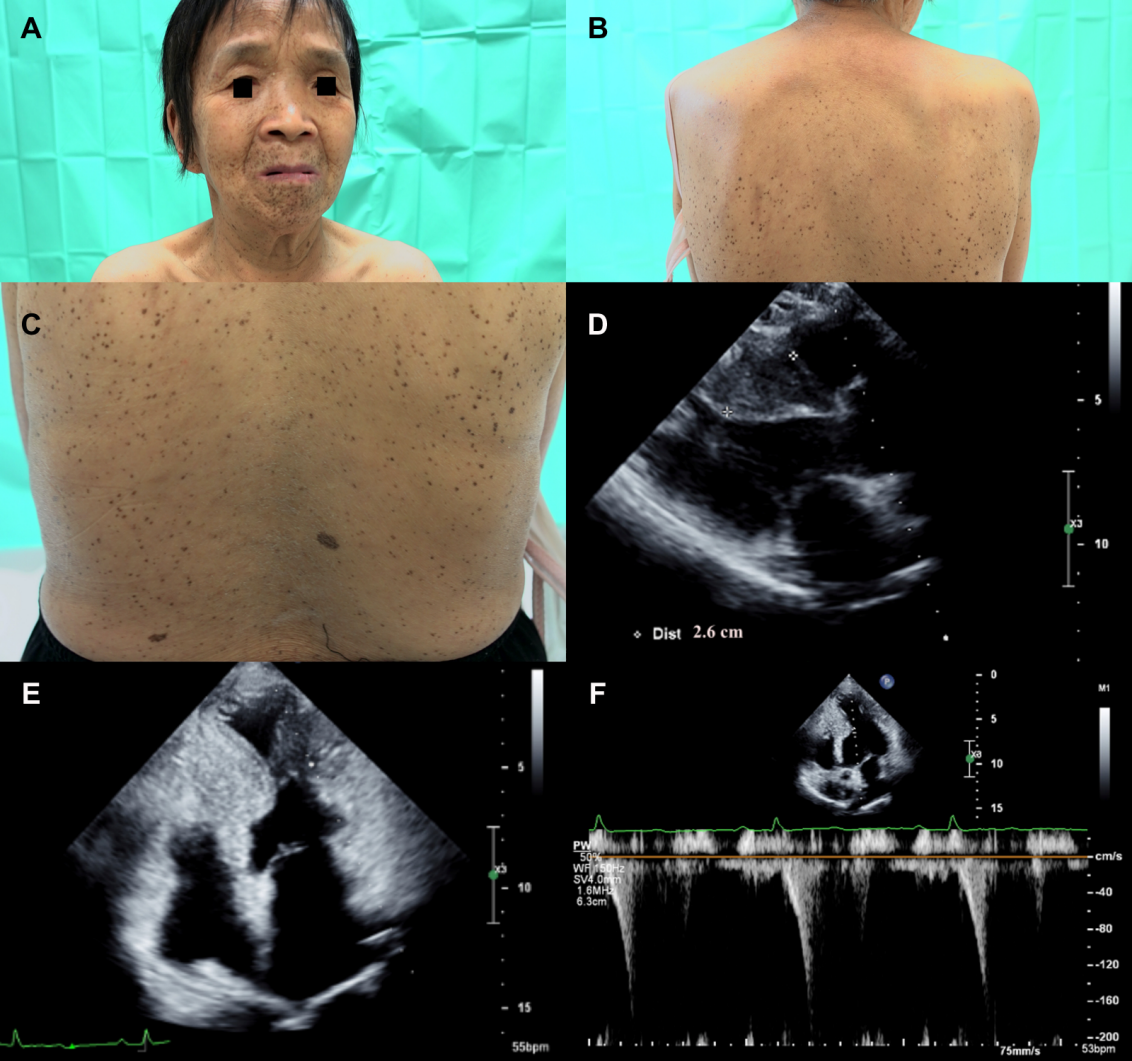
(A) The proband's mother (individual I-8) presented with ocular hypertelorism and diffuse black to brownish facial lentigines. (B) Numerous lentigines on her back, varying in size and shape. (C) Close-up of back lentigines with diameters ranging from 1 mm to 5 mm. Echocardiography revealed (D) significant septal wall hypertrophy (septal wall thickness: 26 mm, cross-mark). (E) Apical aneurysm formation was revealed with midventricular obstruction. (F) Pulsed wave Doppler showed mid-cavity pressure gradient was around 17 mmHg.
